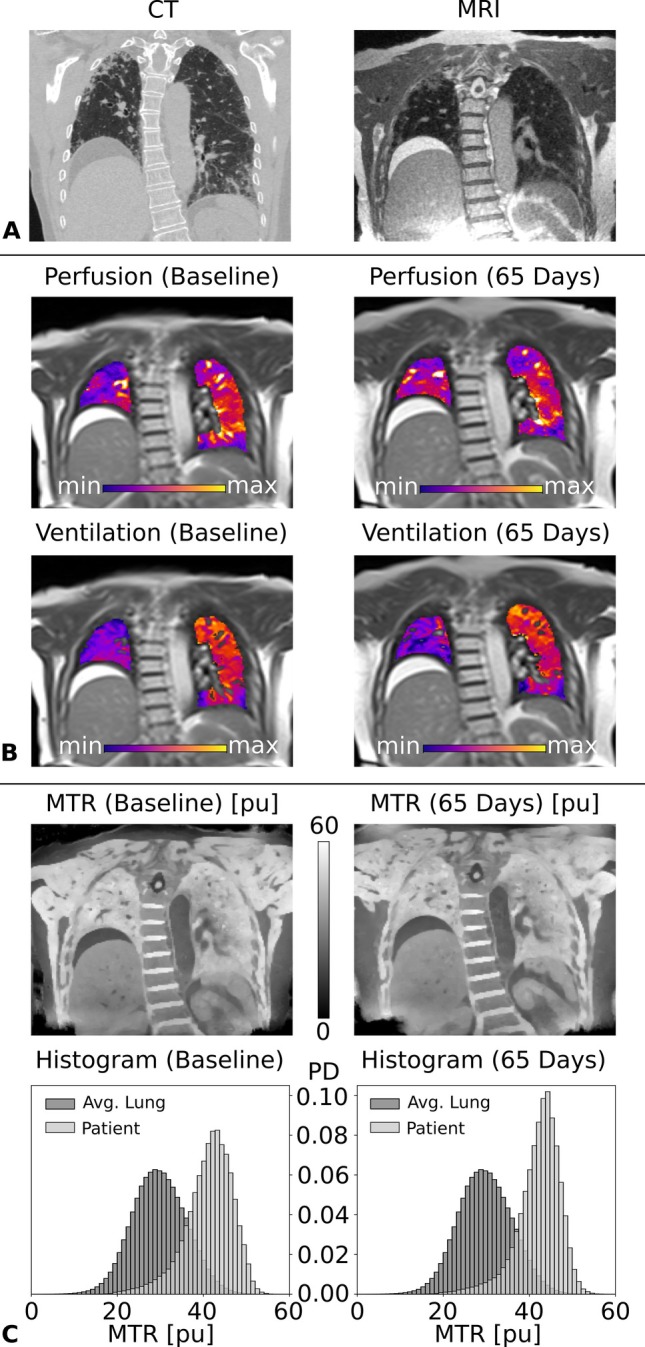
Pulmonary fibrosis patient with acute inflammation: (A) morphological images: CT and MRI, (B) functional maps: perfusion‐weighted MP MRI map and ventilation‐weighted MP MRI map of the first visit on the left and of the second visit after 65 days on the right, and (C) MTR maps and whole lung MTR probability density (PD) histograms in light gray of the first visit on the left and of the second visit after 65 days on the right. For comparison, a histogram representing collective data of all healthy volunteers, representing an average healthy lung, is shown in dark gray.
